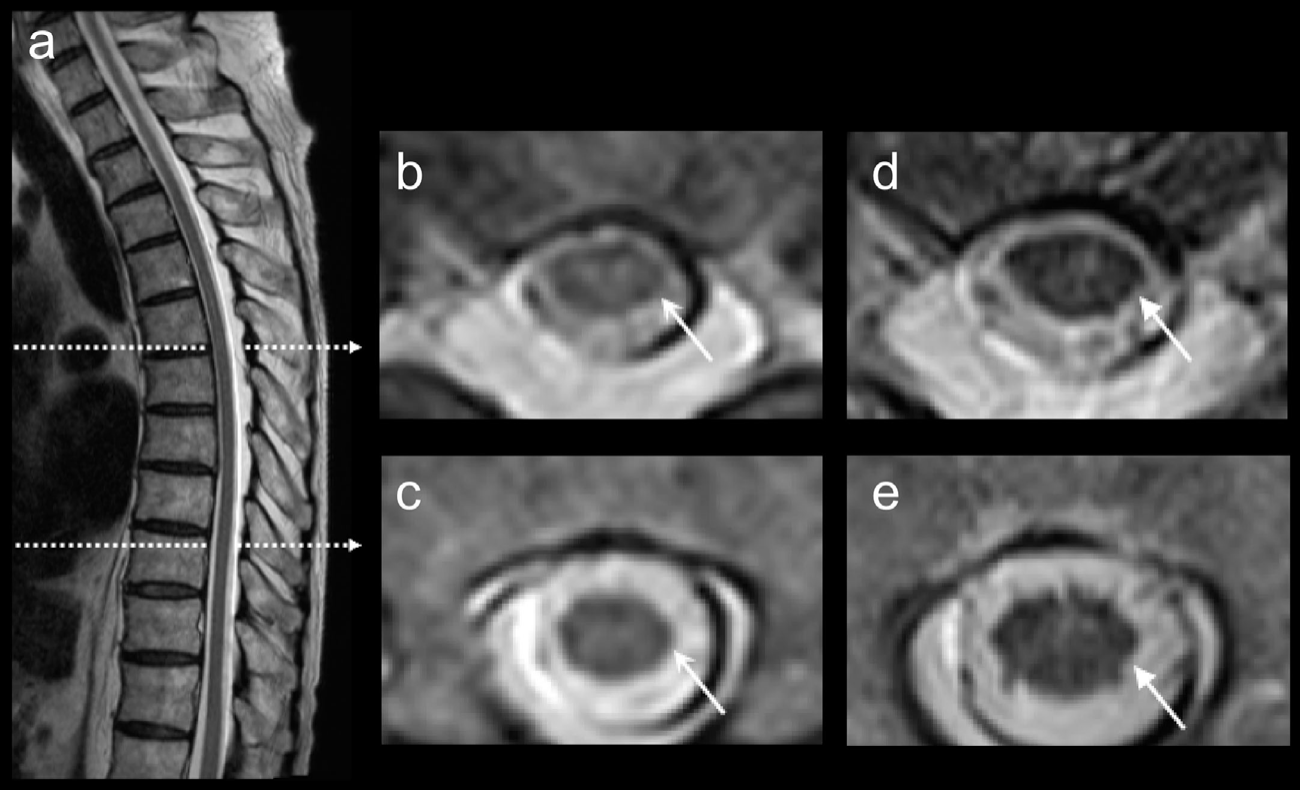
Thoracic magnetic resonance imaging (MRI). T2-weighted thoracic MRI showed abnormal high signals in the spinal cord (A–C). After 5 months, the high signals were diminished on the follow-up thoracic MRI (D,E).
